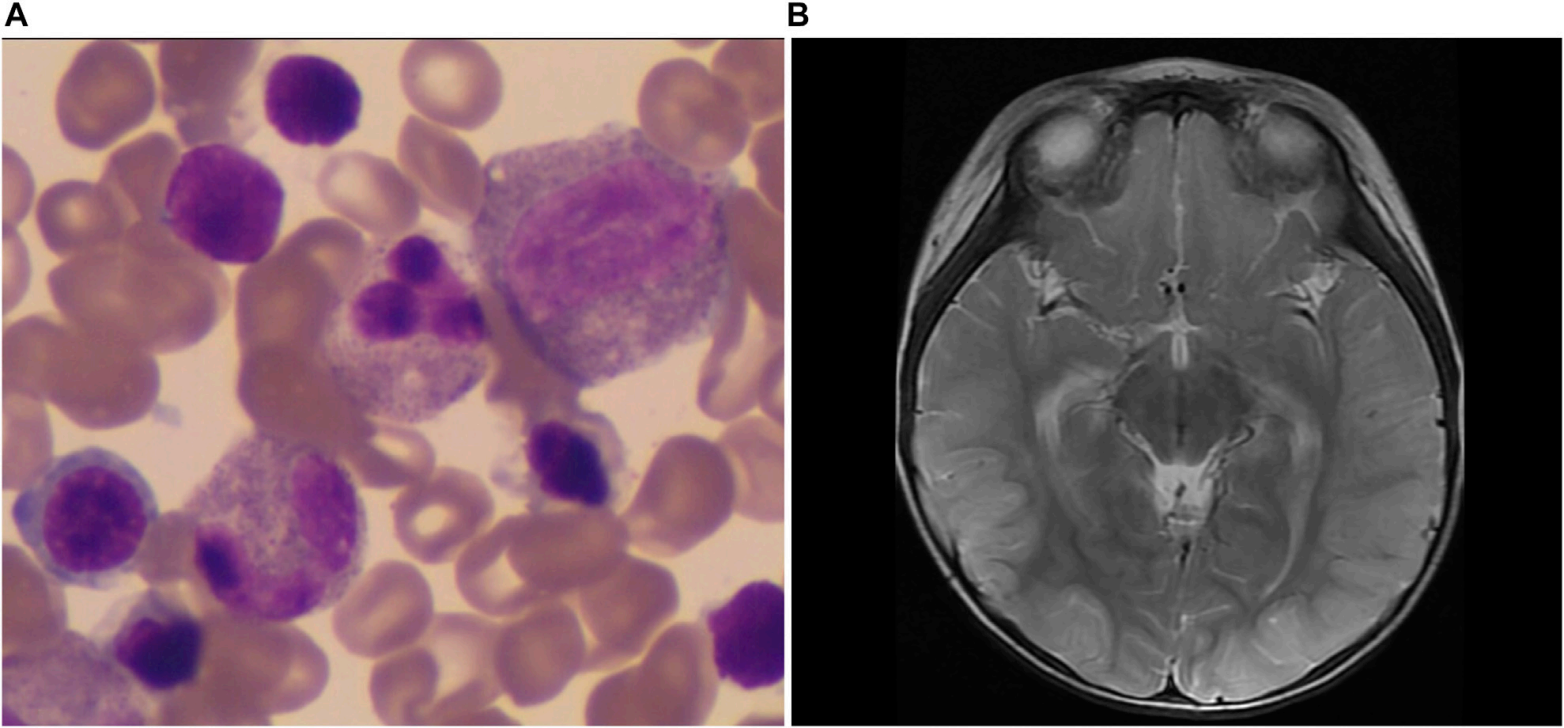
The patient’s main clinical characters. (A) Bone marrow aspirate showed markedly active myeloproliferation with impaired megakaryocyte maturation. (B) The brain DWI image. It showed linear hyperintensity in the left occipital lobe on diffusion-weighted imaging (DWI).
